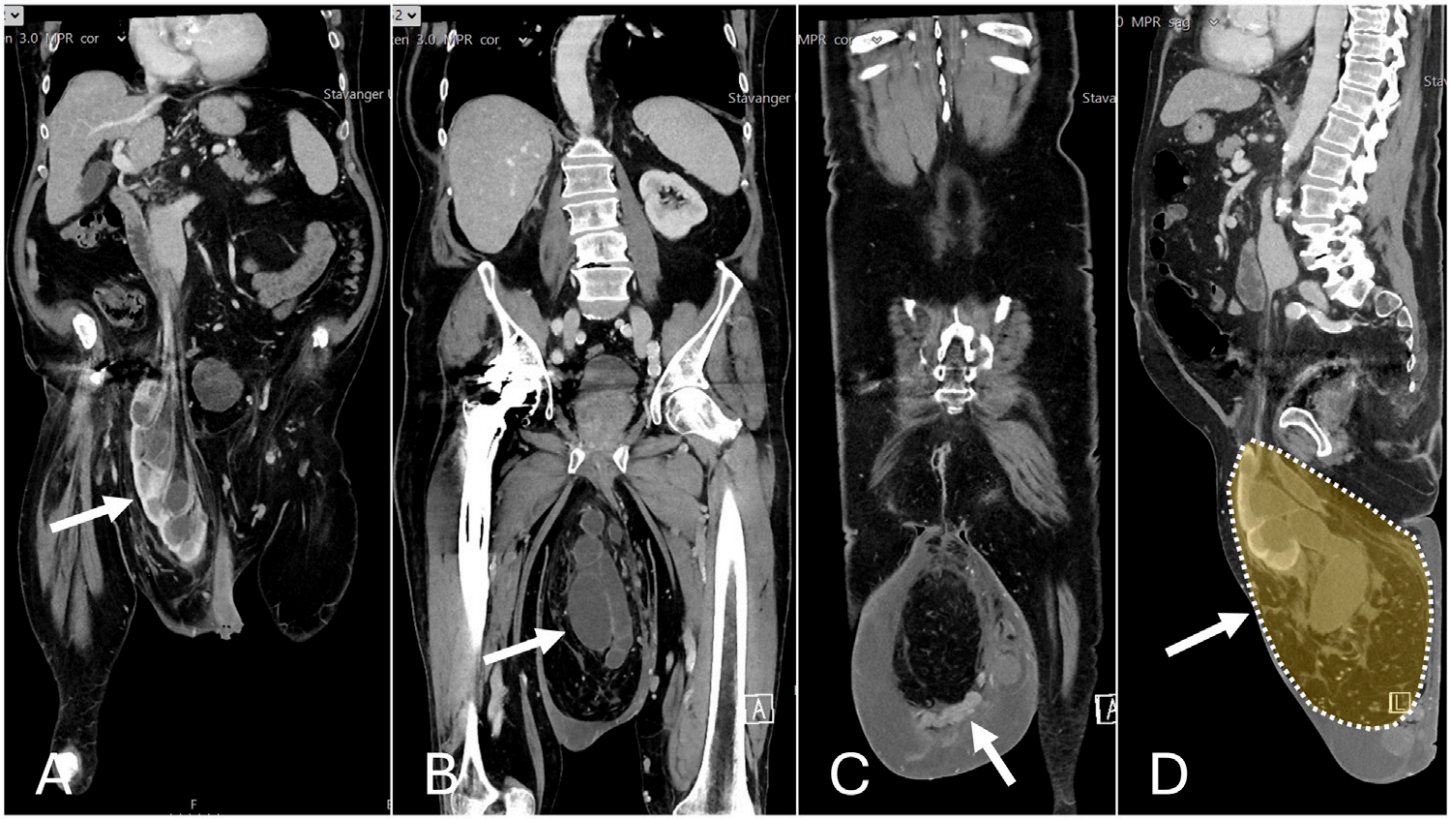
Preoperative CT scan. (A) Incarcerated kidney. (B) Hydroureter. (C) Varicose veins and edema. (D) Loss of domain.
